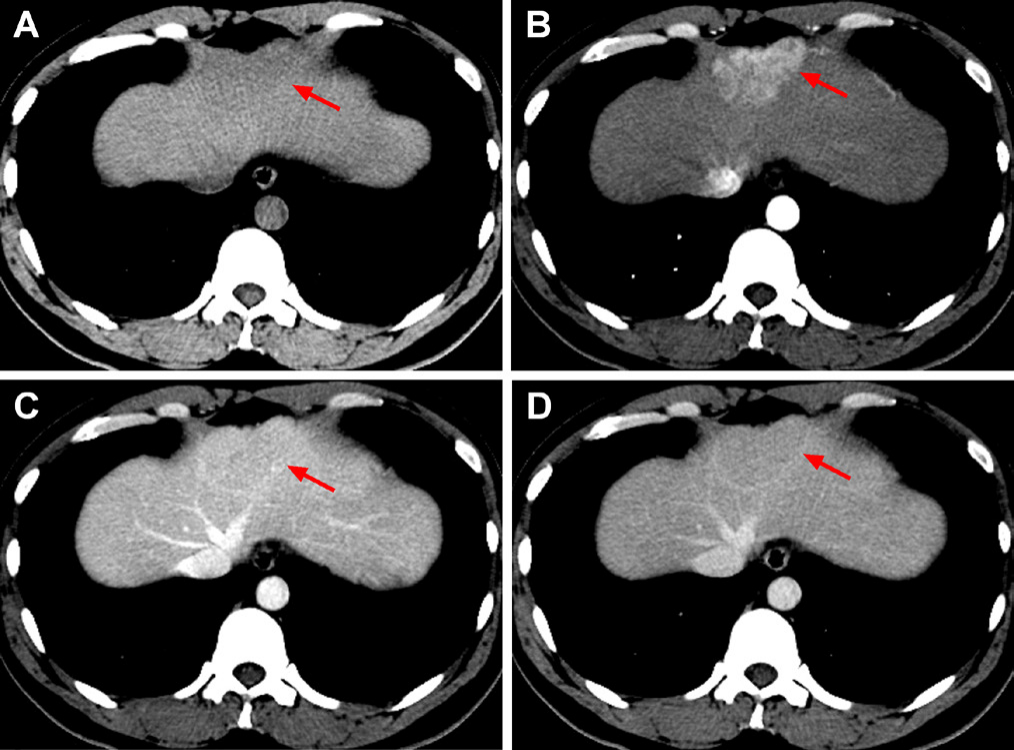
CT images of IHS in case one. Non-contrast CT revealed a round, slightly hypodense mass located in segment II/IV of the left lobe of the liver with an unclear boundary (A, arrow). After contrast injection, the mass exhibited heterogeneous hyperenhancement in the arterial phase (B, arrow), isodensity in the portal venous phase (C, arrow), slight hypodensity in the delayed phase (D, arrow) and delayed enhancement of the capsule (C, D, arrow).
